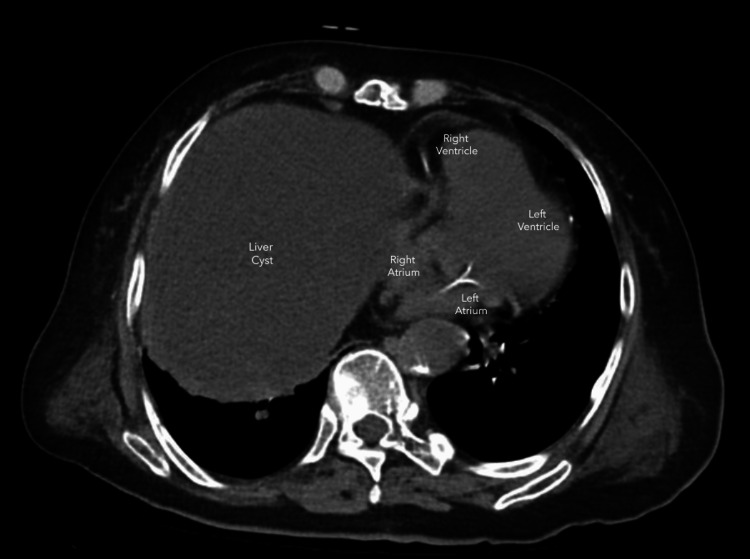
Axial cut of CT images showing extrinsic compression of the right heart by a large simple hepatic cyst
